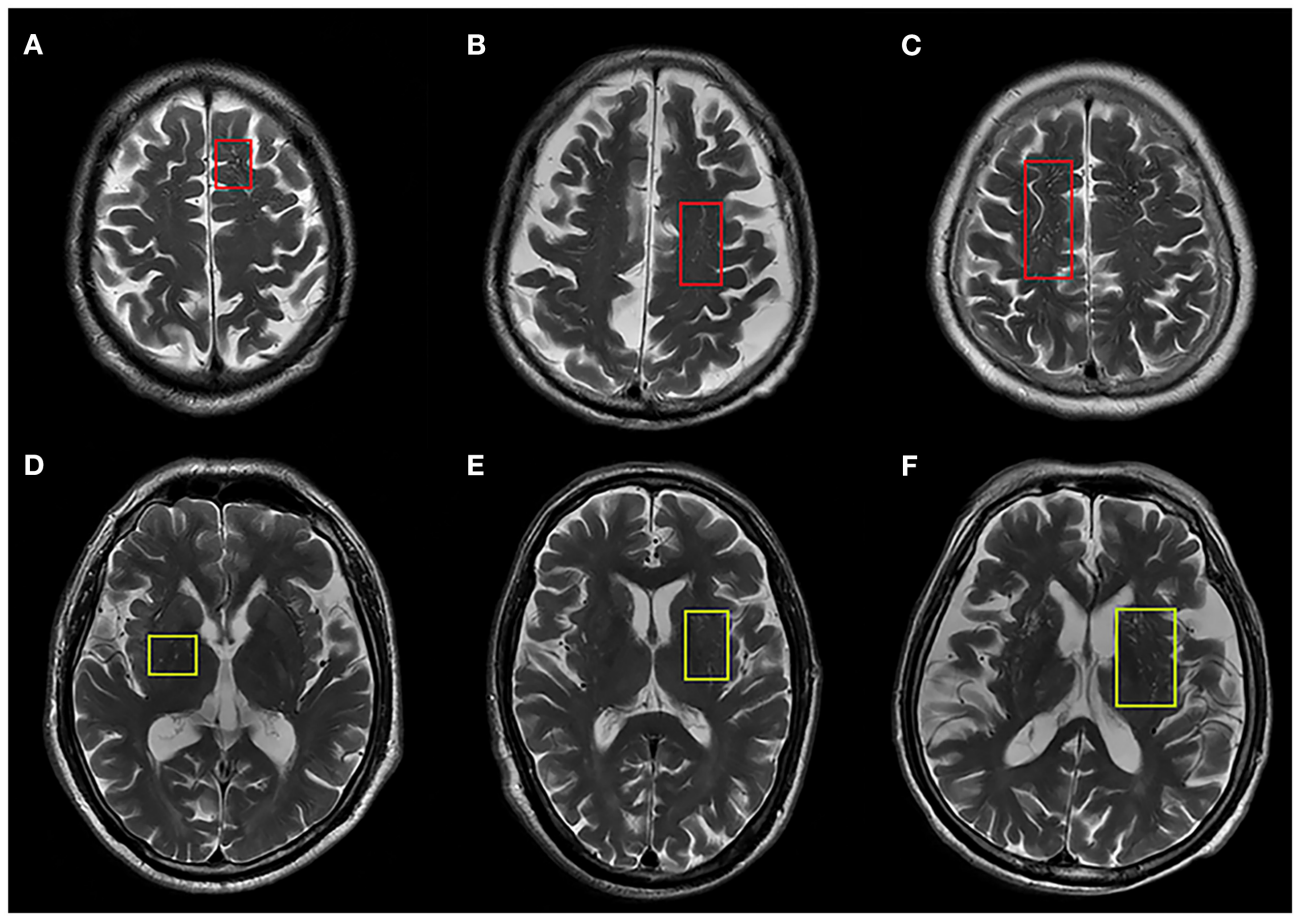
Representative axial T2-weighted images of enlarged PVS in centrum semiovale (ePVS-CSO) and basal ganglia (ePVS-BG). (A–C) Indicate ePVS-CSO with red squares corresponding to degree of severity. (D–F) Indicate ePVS-BG with yellow squares corresponding to degree of severity.
